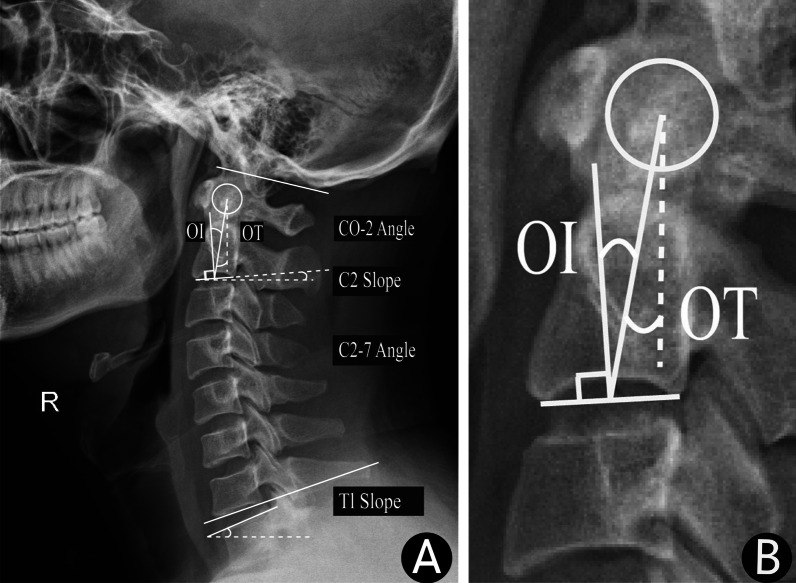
Measurement of odontoid process parameters and cervical spine parameters on DR images. A: Measurement of OI, OT and cervical spine parameters on DR images of a healthy 35-year-old male; B: High-resolution views of OI and OT on DR images of a healthy 35-year-old male.DR, digital radiography; OI, odontoid incidence; OT, odontoid tilt
